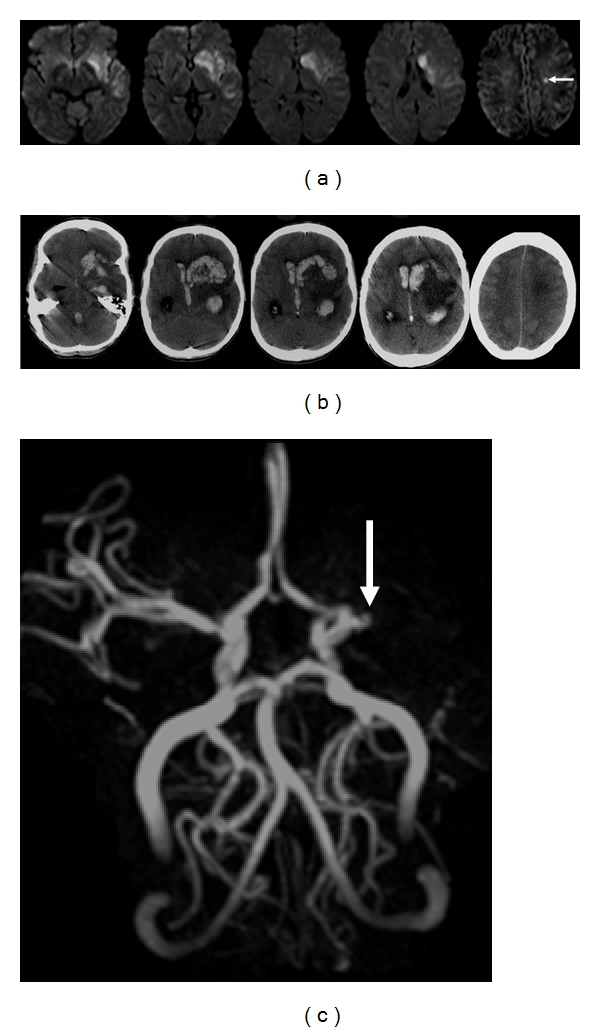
24-year-old woman presented with dense right hemiparesis and seizure. (a) Initial axial diffusion weighted MR images show an extensive area of diffusion restriction in the left basal ganglia and insular cortex. There is also a small area of restricted diffusion in the left corona radiate (arrow). Staphylococcus was grown from her peripheral blood culture, and she was treated for bacterial endocarditis. (b) Axial unenhanced CT images 3 weeks later show extensive hemorrhage within the area of the initial infarction. Stage IIH d2 [1]. (c) MR angiogram shows occlusion of the left middle cerebral artery, consistent with hemorrhagic transformation of the initial stroke.
